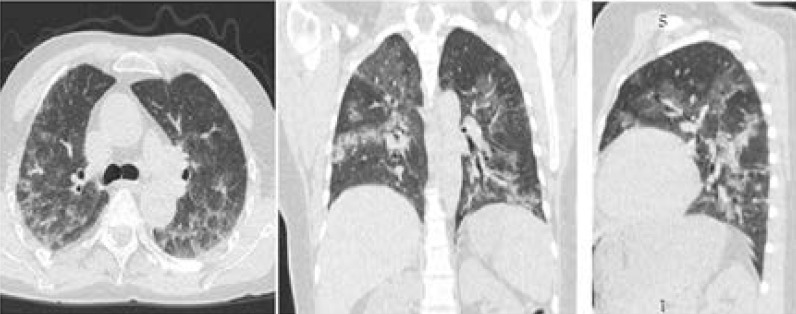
13 days after follow-up (21/4/2020), Chest CT non-contrast in axial, coronal and sagittal pulmonary parenchymal show reduced size and density of the lesions.
